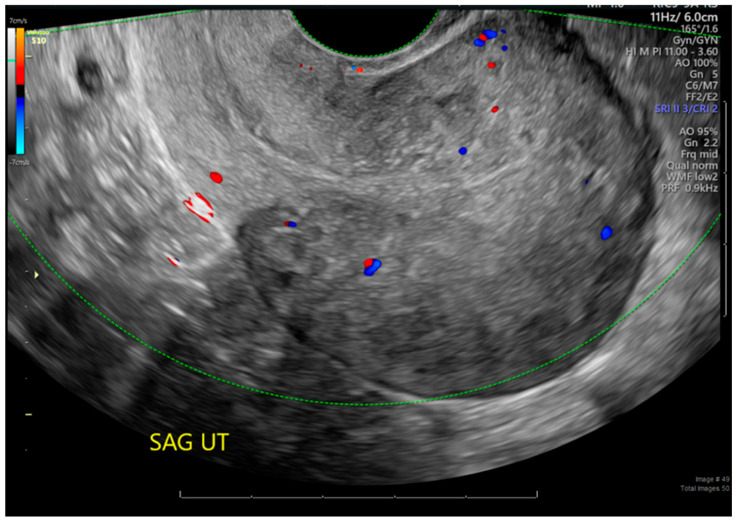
Two-week follow-up ultrasound showing endometrial width of 7.1 mm without abnormal Doppler flow, indicating resolution of accreta with Sonata-guided ablation.
